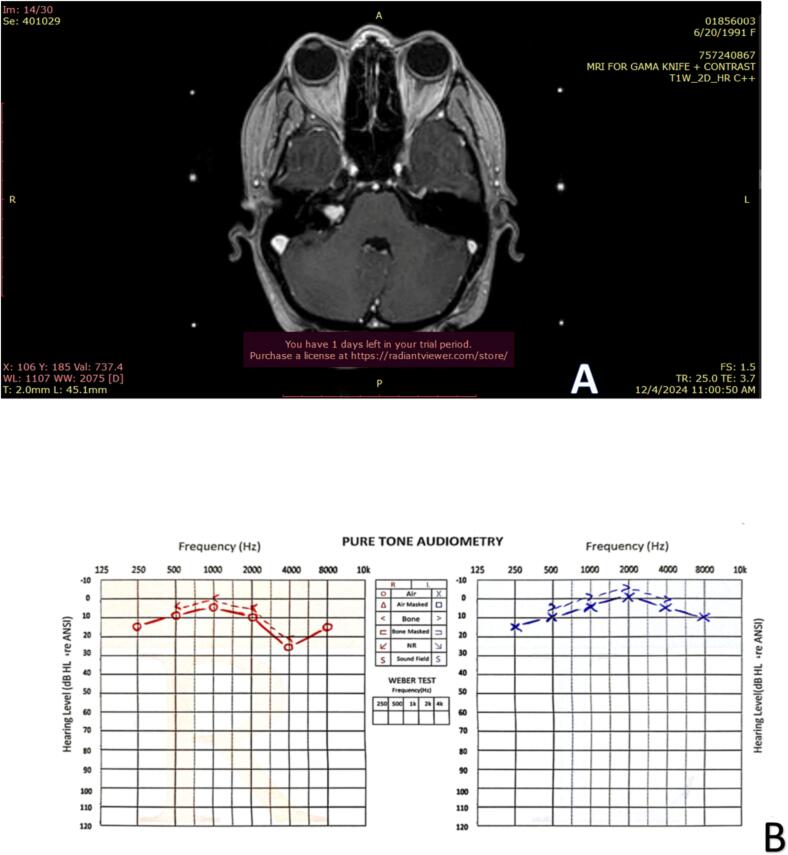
Case 1. A: Magnetic resonance imaging (MRI) revealed a 1.2 × 0.6 × 0.6 cm tumor in the right cerebellopontine angle (CPA). B: Pure Tone Audiometry revealed a mild high-frequency hearing loss in the right ear (10, 15 dB on 6, 8 K Hz respectively).
